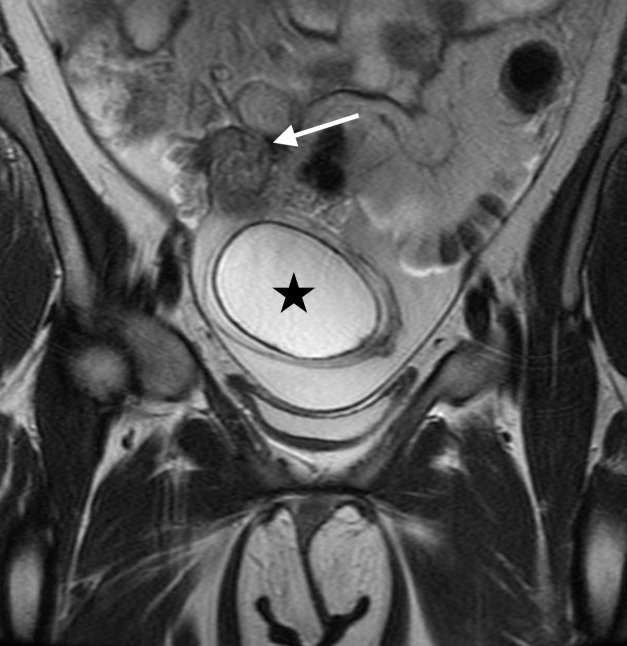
Coronal T2 weighted magnetic resonance imaging of the pelvis demonstrates a 7-centimeter fluid signal tubal mass (star) with a twisted appearance of the torsed right fallopian tube (arrow).
